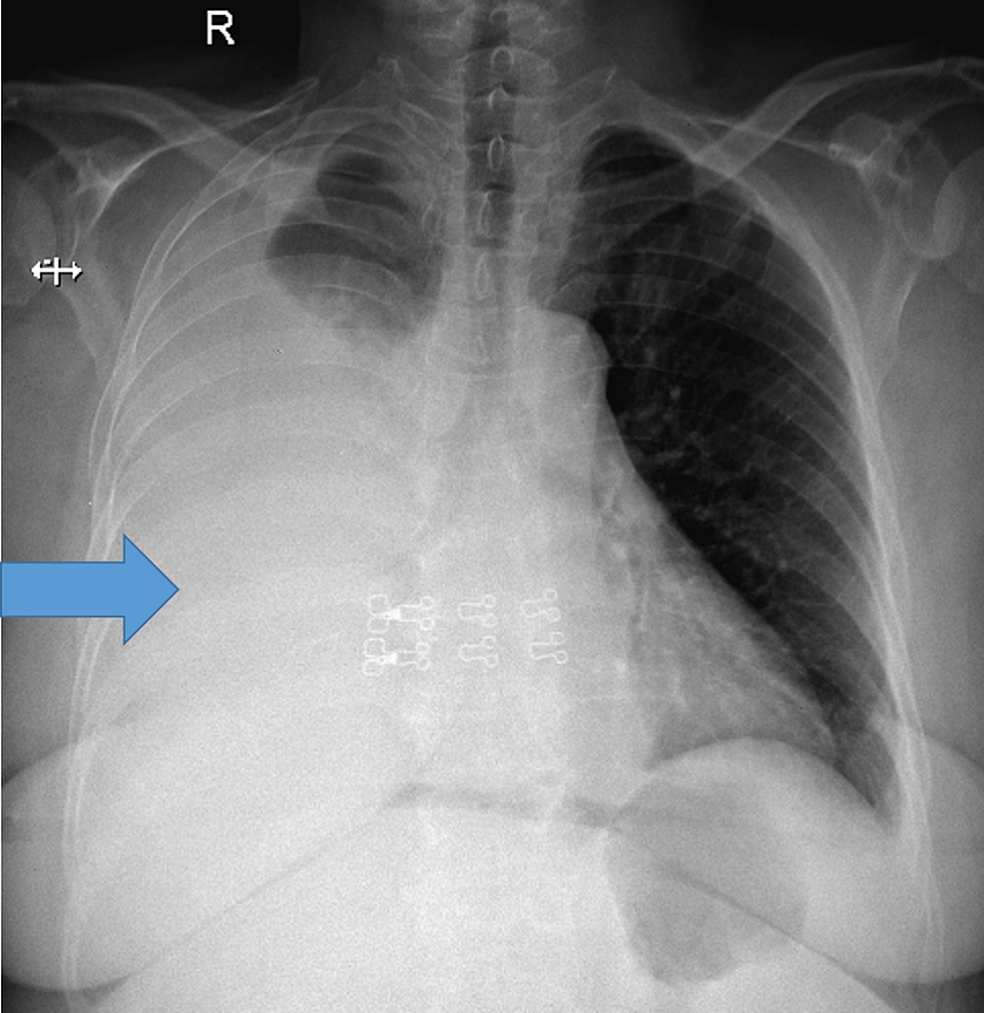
Chest radiograph showing massive right pleural effusion
